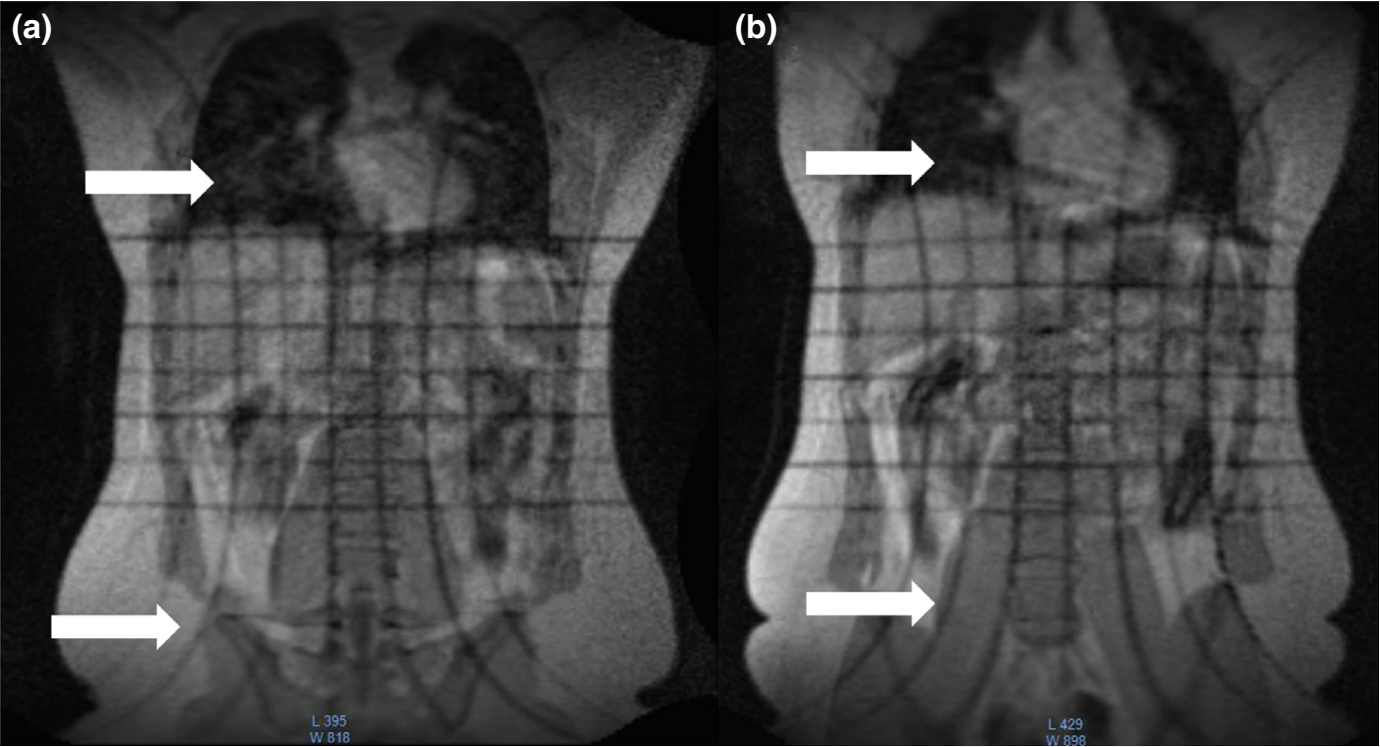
2D T_1_‐weighted gradient echo coronal MRI localizer (TE/TR: 3.44/147 ms, 2 × 2 × 5 mm, gap: 25 mm, flip angle: 60^0^, 300 Hz/pixel, 7 slices/orientation, 28 s) showing axial and sagittal (arrows) slice excitation profiles *in vivo*. Gradient nonlinearities cause the slice profiles to curve away from isocenter at large offsets from isocenter. The nonlinearities are comparable between the MRI‐^60^Co (a) and the MRI‐Linac (b). The gradient nonlinearities emphasize the importance of placing the target as close to isocenter as practical to minimize their effect on geometric accuracy. MRI, magnetic resonance imaging.
